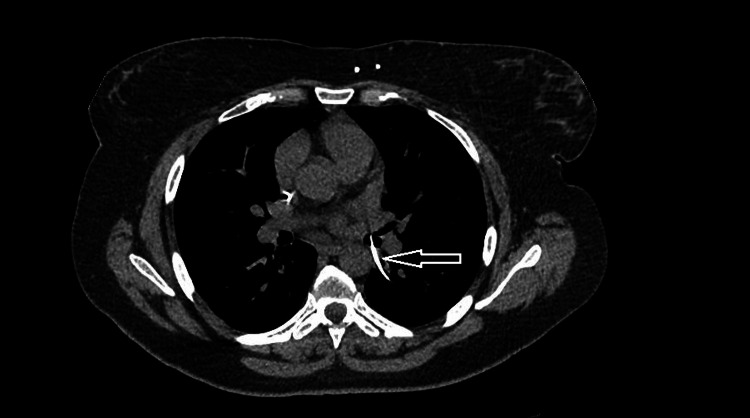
CT scan showing the stent in the right lower lobe CT: computed tomography
